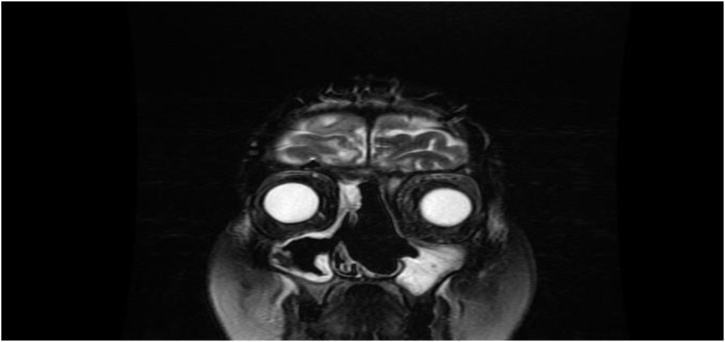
Postoperative and adjuvant radiotherapy, MRI T2.
